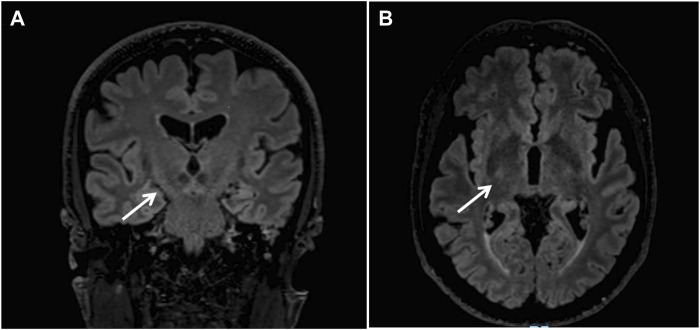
Brain Magnetic Resonance revealed bilateral fronto-parietal atrophy; coronal (A) and axial (B) FLAIR-T2-weighted sequences displayed hyperintensity of the cortico-spinal tracts (arrows).
